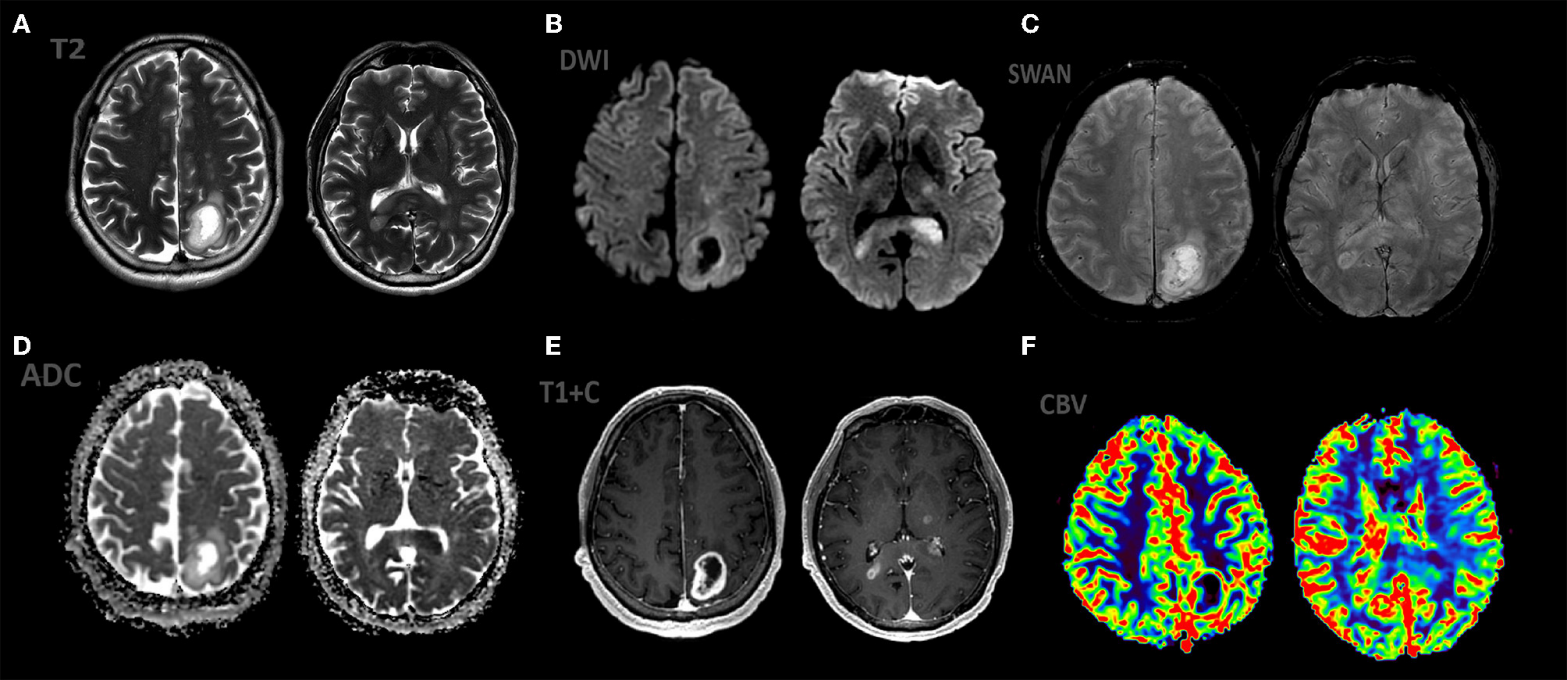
Patient S., male 55 y.o. presented with right sided weakness and memory loss. MRI ax: (A) T2WI; 6, (B) DWI, (C) ADC, (D) SWAN, (E) T1 + C, (F) DSC-PWI. Axial Brain MRI: there is a intraaxial mass in left parietal lobe with low peritumoral edema on T2WI, another mass in the splenium of the corpus callosum and left thalamus. All mass parts have restricted diffusion and bright, homogeneous contrast enhancement and hight rCBV values.
